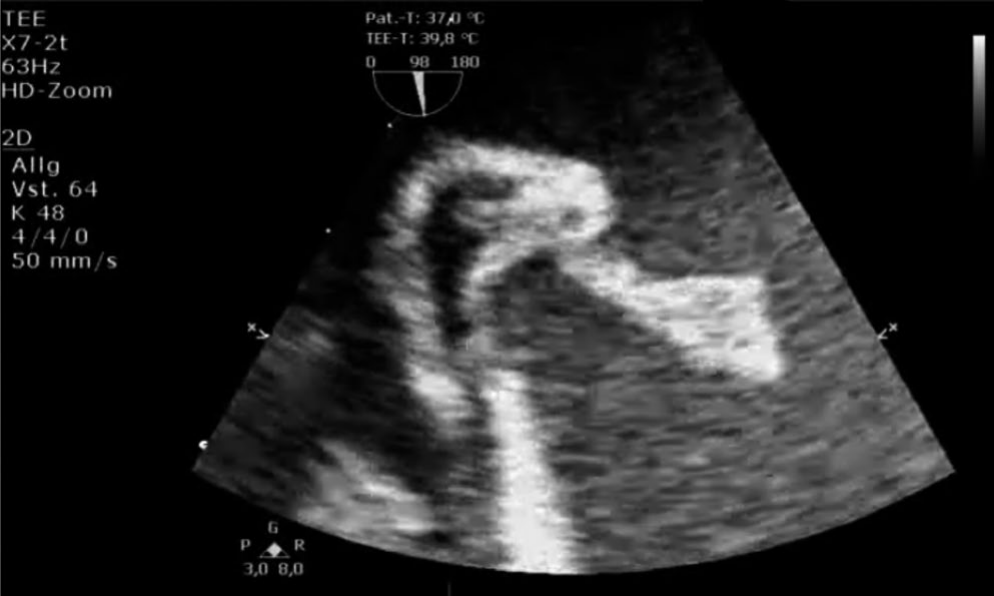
Transesophageal echocardiography: focussed midesophageal short‐axis view of the mobile, tubular structure in the right atrium. A focussed midesophageal short‐axis view of the right atrium showed the echogenic, mobile, tubular structure at the transition of the inferior vena cava into the right atrium. It measured 4.1 cm. Although being of relative high echogenicity and atypical location, in the context of positive blood cultures and intravenous drug abuse, it was highly suspect of a vegetation. An additional movie file is included, showing the movement of the tubular structure (see Video S3)
